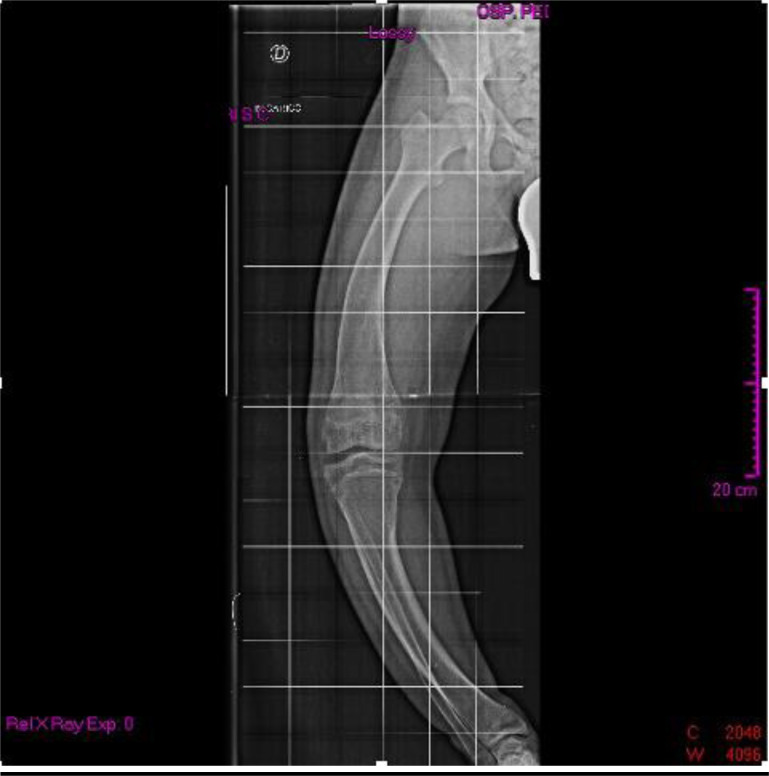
X-ray evaluation of the right leg.
